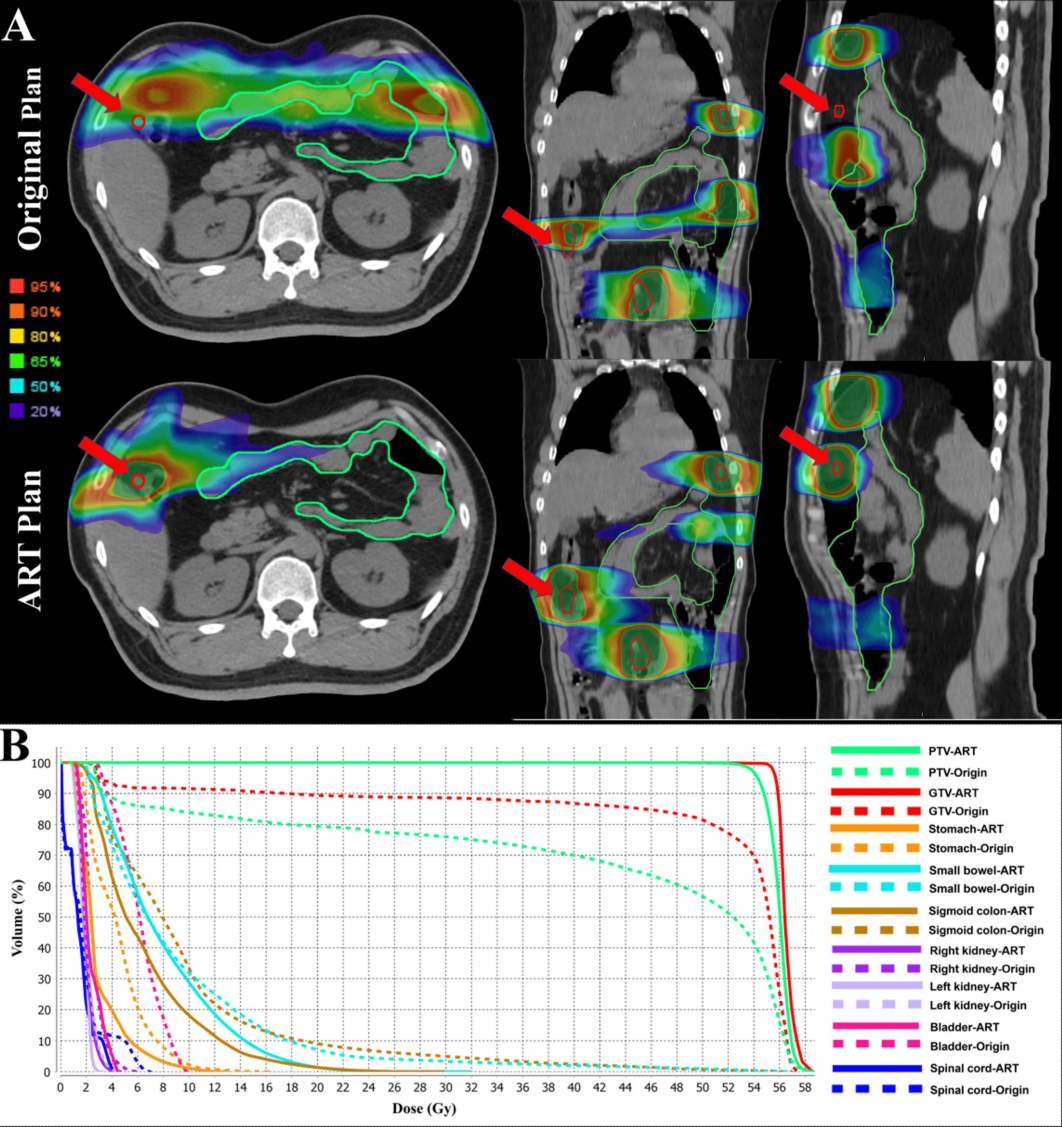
The superiority of FBCT-gART over nART The displayed images are acquired from the initial fraction of radiotherapy, preceding the first daily FBCT scan, and show axial, coronal, and sagittal views (A), the red line outlines the GTV, and red arrows mark the areas of GTV coverage by the ART plans and GTV misses by the nART plans. The DVH curves (B) provide a quantitative comparison between the ART and nART plans, as shown by the solid and dotted lines, respectively. *Abbreviations:* ART: Adaptive radiotherapy; nART: Non-adaptive radiotherapy; FBCT: Fan beam computed tomography; GTV: Gross tumor volume; PTV: Planning target volume; DVH: Accumulated dose-volume histogram
